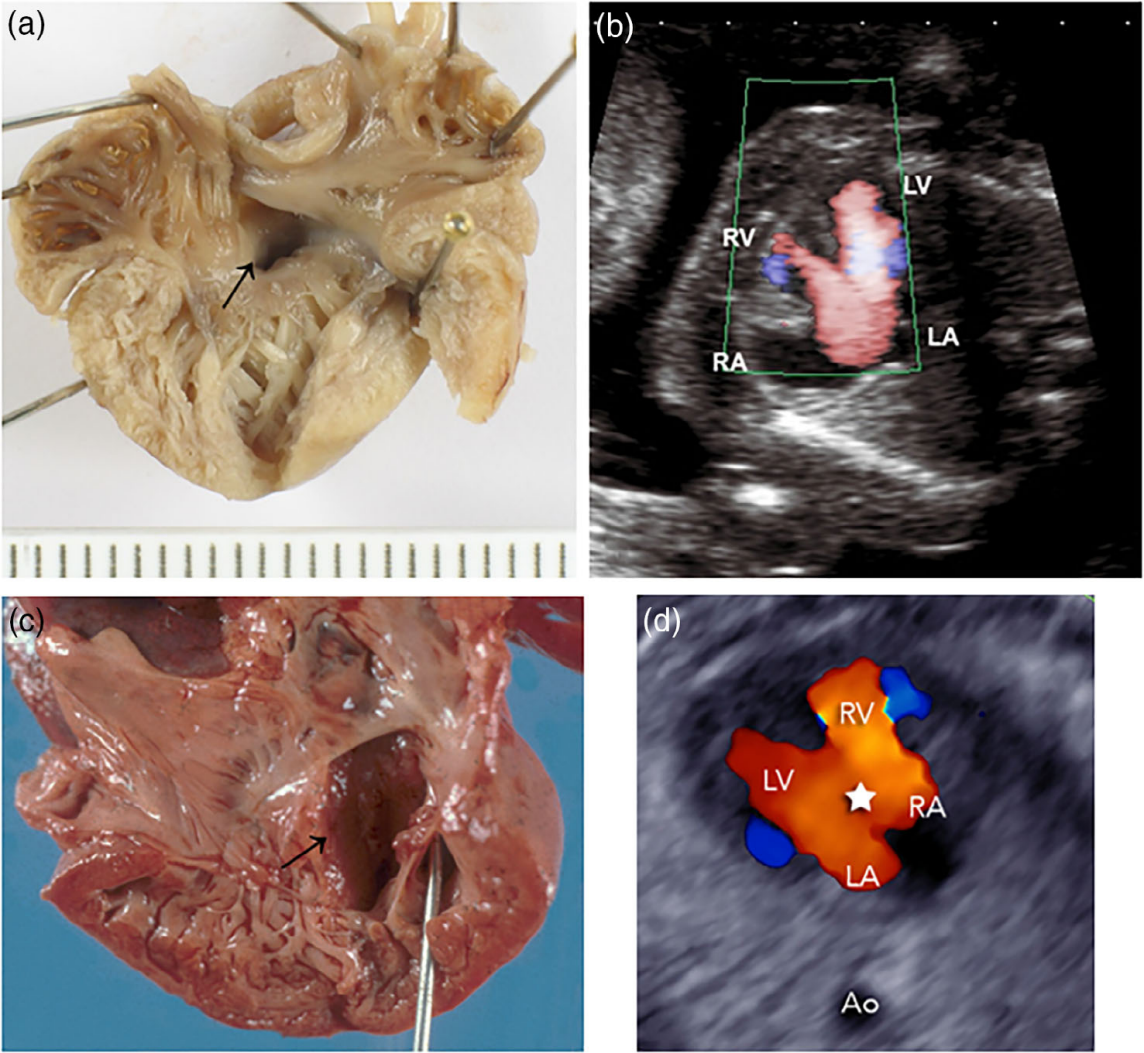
Cardiac inflow tracts of the right ventricles showing partial atrioventricular canal defect (AVSD) with ASD1 (→), minimal upper VSD and tricuspid valves communicating with the mitral valves in Case 7 (a) and complete AVSD with ASD1, large VSD (→) and common atrioventricular valves in Case 9 (c). Corresponding prenatal Doppler sonography of Case 7 (b) and Case 9 (d)
